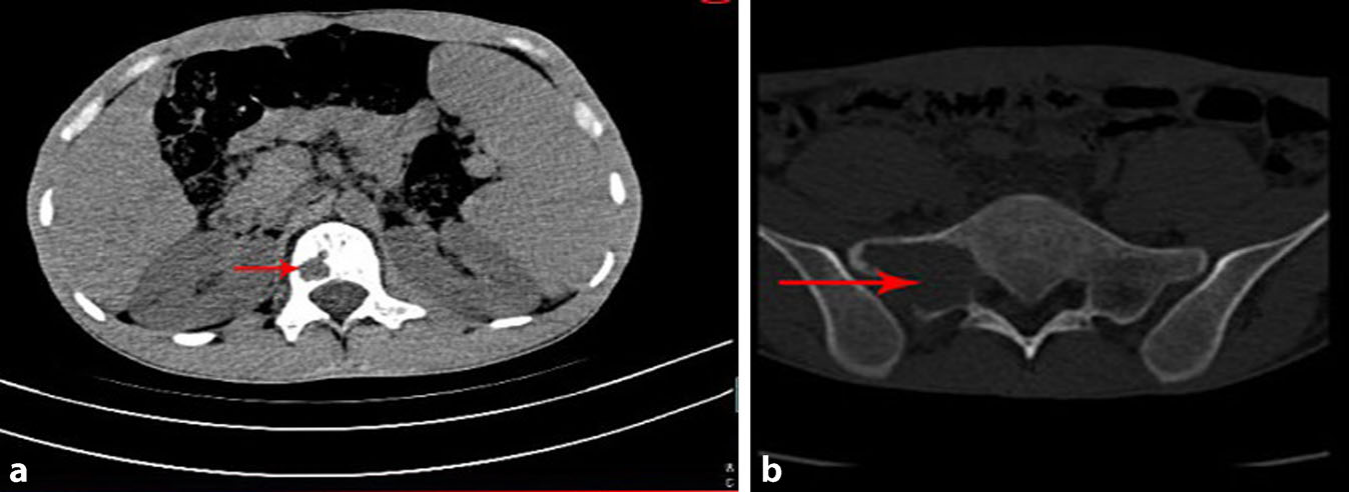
Preoperative computed tomography of S1 and L1 reveals bone destruction and vertebral abnormalities. a L1 Vertebral lesions and vertebral invasion. b S1 Vertebral lesions and vertebral invasion. *Red arrows* indicate location and extent of the lesions
